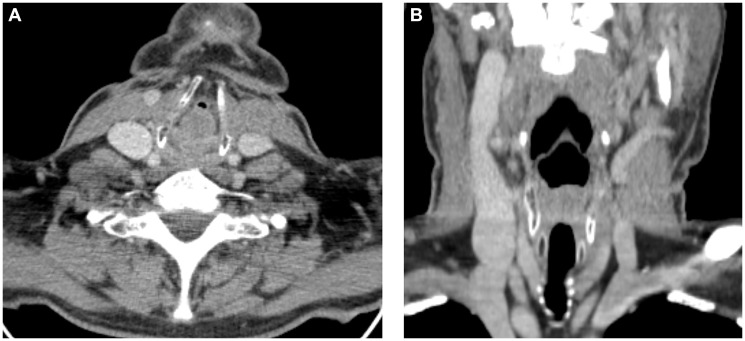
CT images in the axial (A) and coronal (B) planes demonstrate a tumor formation of the larynx, subtotally filling the lumen of the larynx.
